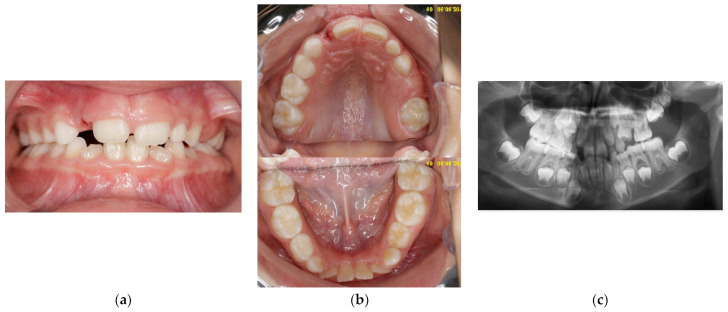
Age of 7 y and 10 m. (a) Frontal view showing slight shift of the upper midline to the left and lower crowding; (b) occlusal view of both arches: 65 fully erupted, with atypical crown shape and size; (c) panoramic X-ray showing impacted 64, bimaxillary crowding, and a tendency for re-inclusion of 74.
